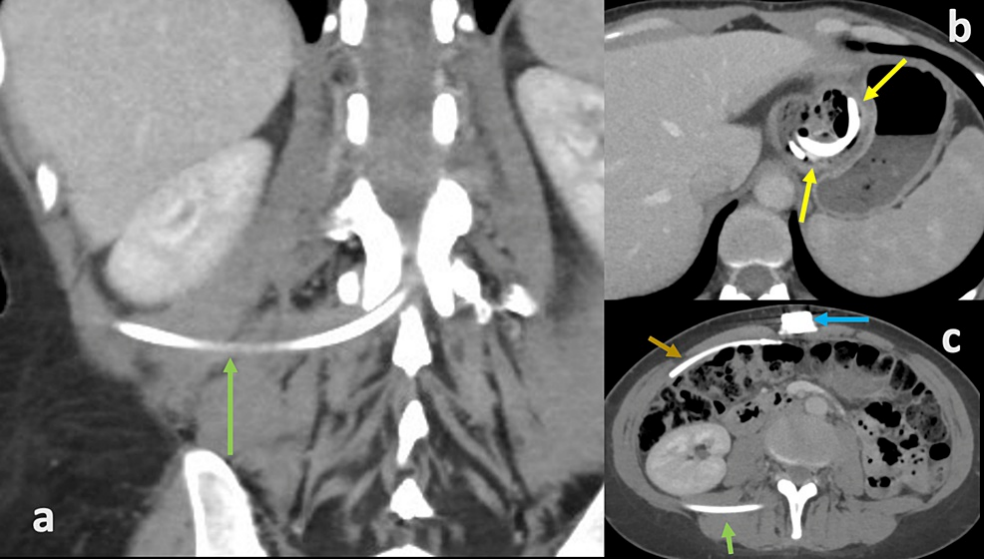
Abdomen/pelvis CT with contrast. Axial (a) and coronal (c) CT images show the catheter fractured (orange arrow) approximately 6 inches from the access port (blue arrow). The catheter segment attached to the band (green arrows) courses through the peritoneal cavity into the right lateral body wall and into the L1-2 disc space. Axial imaging (b) reveals erosion of the gastric band through the stomach wall and into the stomach lumen (yellow arrows).
